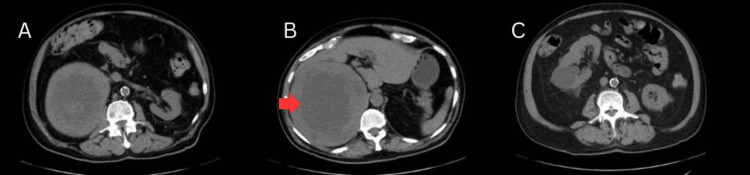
Axial CT image of a 17-cm adrenal hemangioma demonstrating a region of central infarction. Axial CT images of the abdomen demonstrating a 17-cm heterogeneous mass arising from the right retroperitoneum (A-B). The mass displaces the right kidney anteriorly and demonstrates central low attenuation with peripheral enhancement (C), features concerning for malignancy such as adrenocortical carcinoma or necrotic sarcoma. An area of central hypoattenuation is noted by the red arrow, which was later determined to be an area of infarction.
